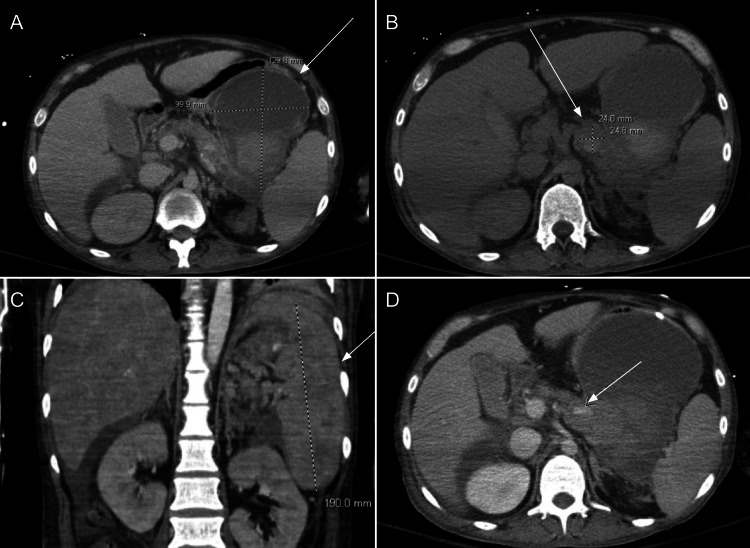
CT abdomen and pelvis with and without contrast on day 13 of hospitalization demonstrated a pancreatic tail hematoma and pseudoaneursym CT abdomen and pelvis with and without contrast on day 13 of hospitalization showed an enlarging pancreatic tail hematoma (10 cm width, 19 cm height, 13 cm length) (A and C). A 2.5 cm pseudoaneurysm in the pancreatic body had slightly increased in size with a developing thrombus that opacifies approximately 40% of the pseudoaneurysm (B). Hypodensity in the pancreatic tail region is consistent with acute necrotizing pancreatitis (D).
